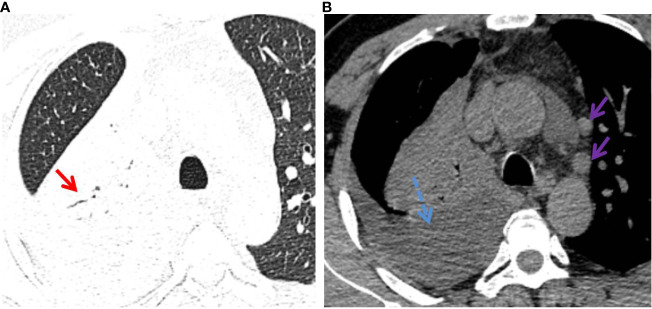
P-LADC in a 70-year-old man without symptoms. (A) Axial HRCT of the lung window showed a consolidation with air bronchogram (red arrow) in the right upper lobe. (B) Mediastinum window showed right pleural effusion (blue dashed arrow) and multiple enlarged lymph nodes in the mediastinum (purple arrow). HRCT, high-resolution computed tomography; P-LADC, pneumonic-type lung adenocarcinoma.
